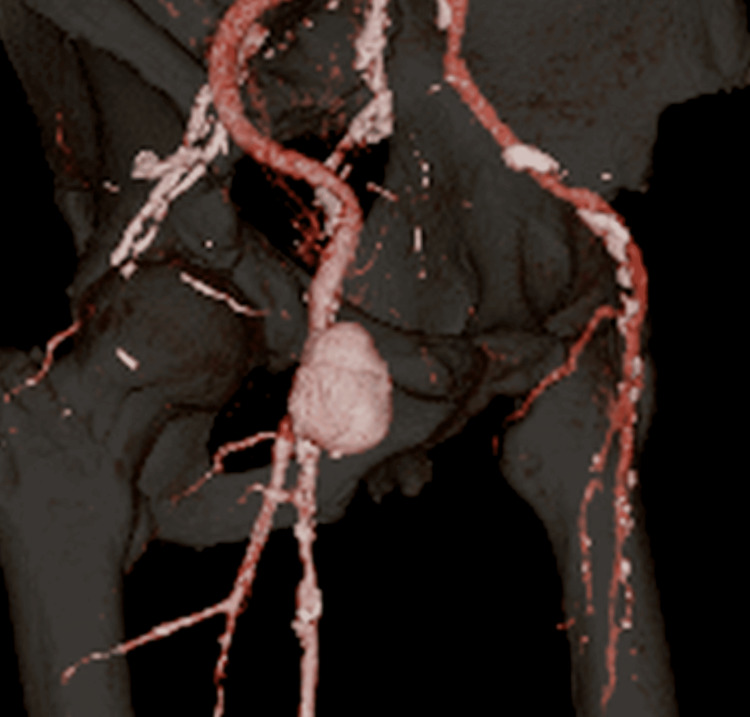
Three-dimensional reconstructed computed tomography image showing the morphology and spatial extent of the pseudoaneurysm arising from the right femoral artery
